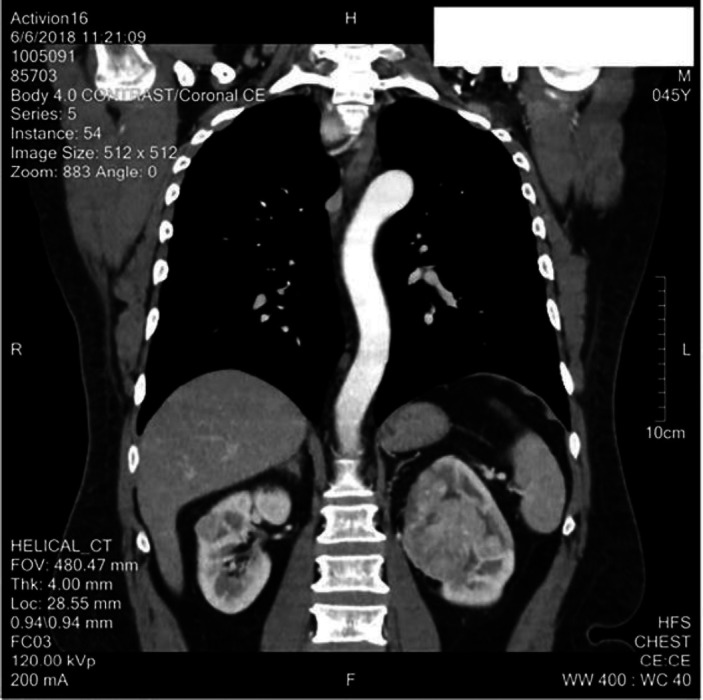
Coronal section of the CT showing renal left renal mass involving upper and mid pole
